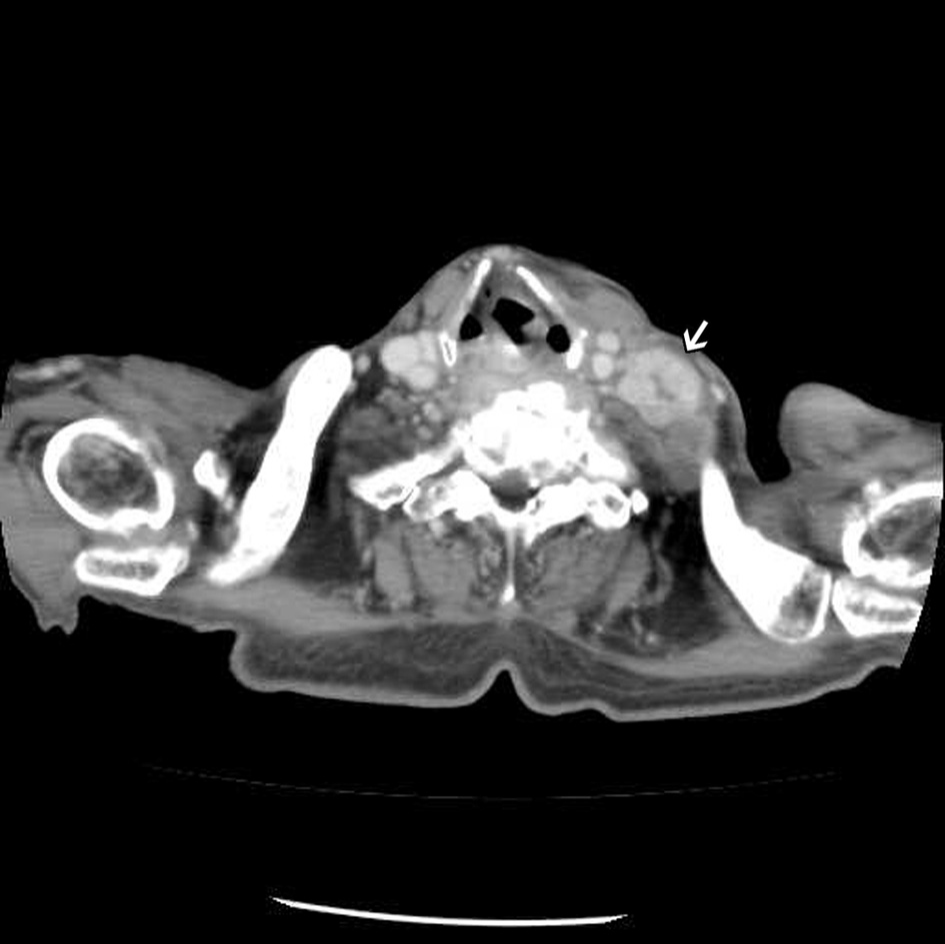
Enhanced chest CT showing an enlarged lymph node at left lower neck.
